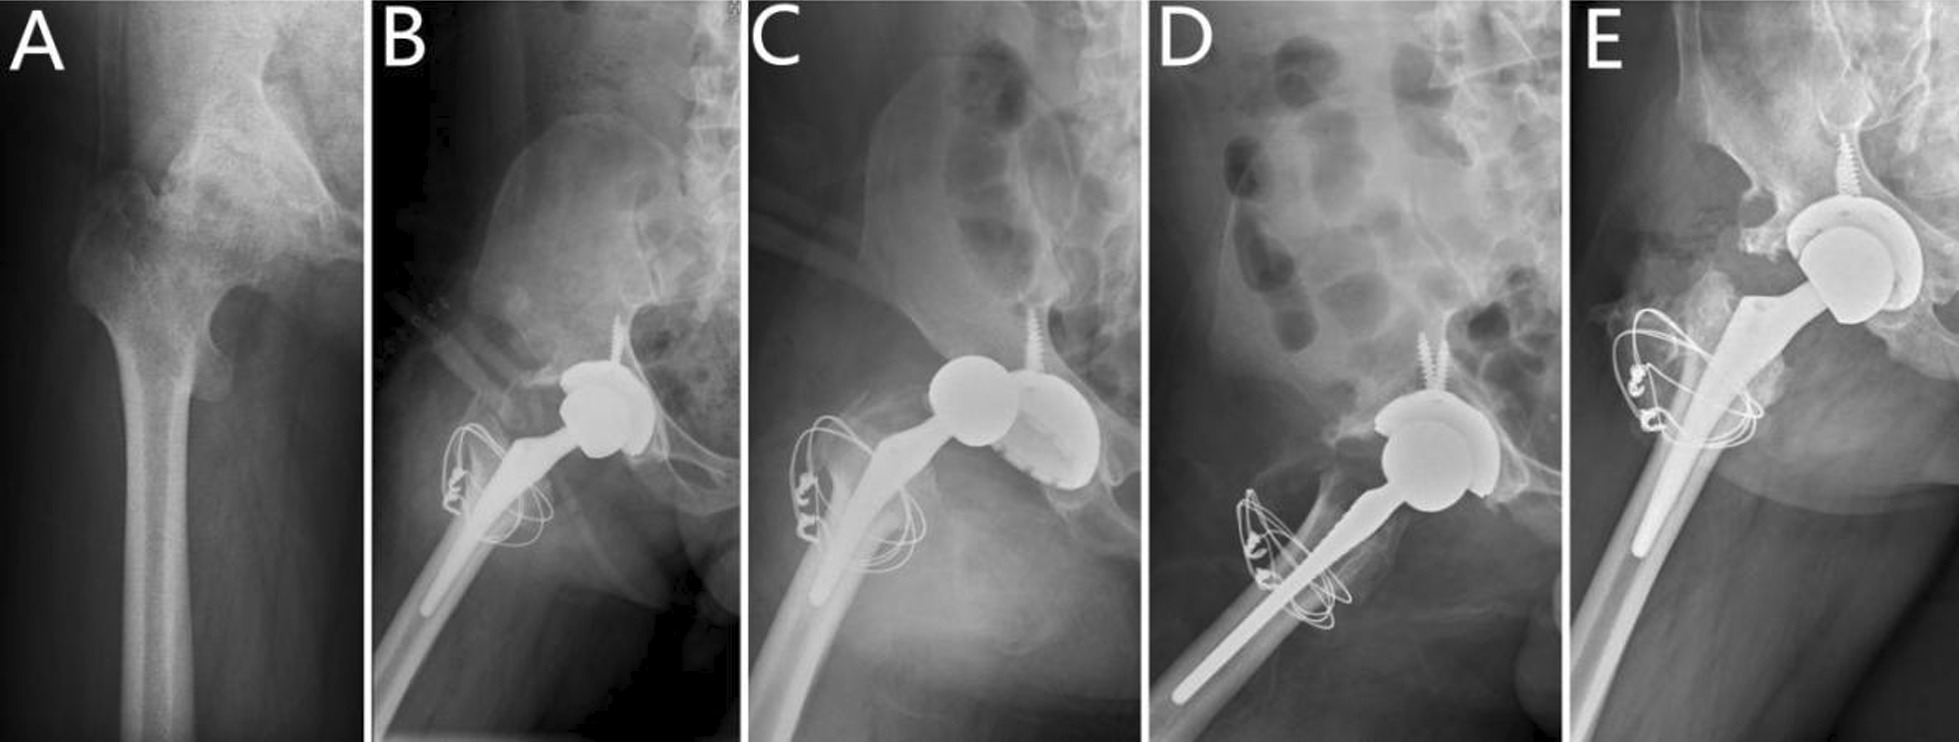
A Preoperative radiograph of the hip of a 43-year-old male with residual poliomyelitis,showing a osteoarthritis secondary to developmental dysplasia of the hip. B Postoperative radiograph. CRadiograph obtained 1 month after surgery showed dislocation of the hip. D Radiograph obtained after the manual replacement on the dislocation of the hip. E At 5.4-year follow-up,radiograph showed a classII heterotopic ossification(Brooker classification system),no evidence of implant subsidence, implant loosening or osteolysis, no dislocation recurred
